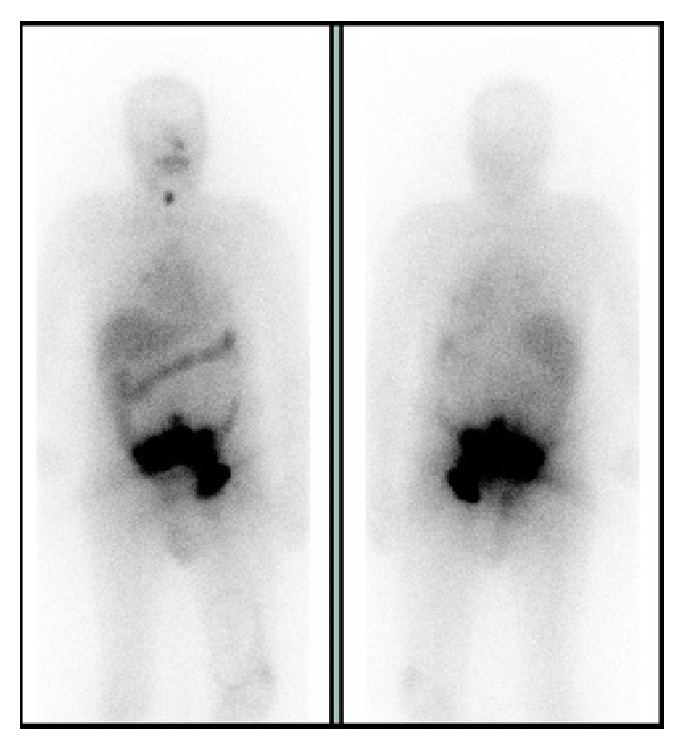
Posttherapy scintigraphy showing hyperfixation in the remnants of the sacrum and lower lumbar spine, bilateral iliac bone, and anterior cervical region [Case 1].
